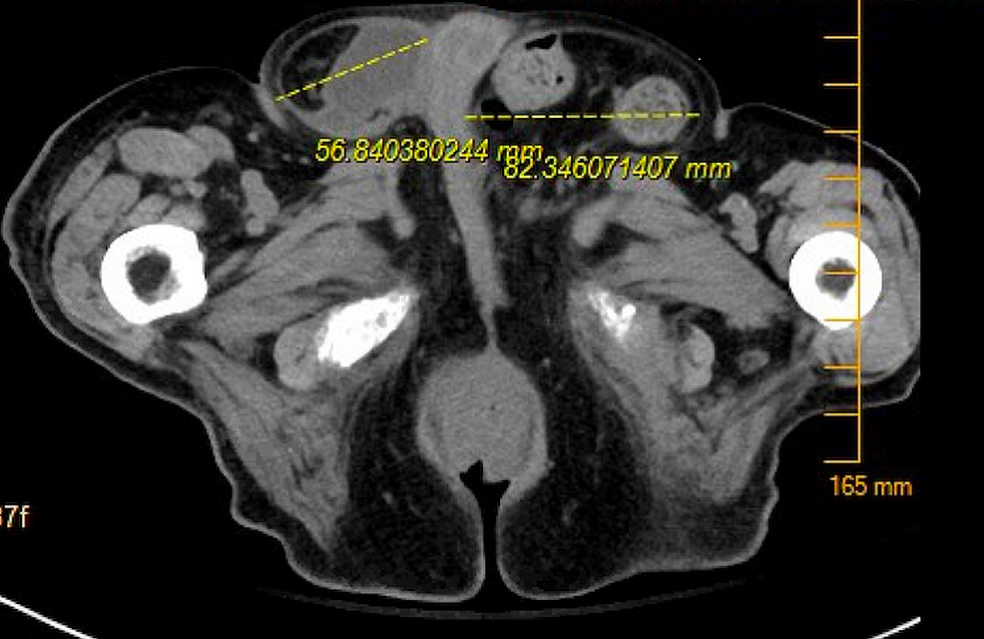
Computed tomography (CT) scan done at the time demonstrated a large left-sided hernia containing the sigmoid colon without evidence of stranding, inflammation, or obstruction. The right side was also noted to have a large hernia with the cecum and appendix in the sac associated with stranding and some pericolonic fluid collection.
